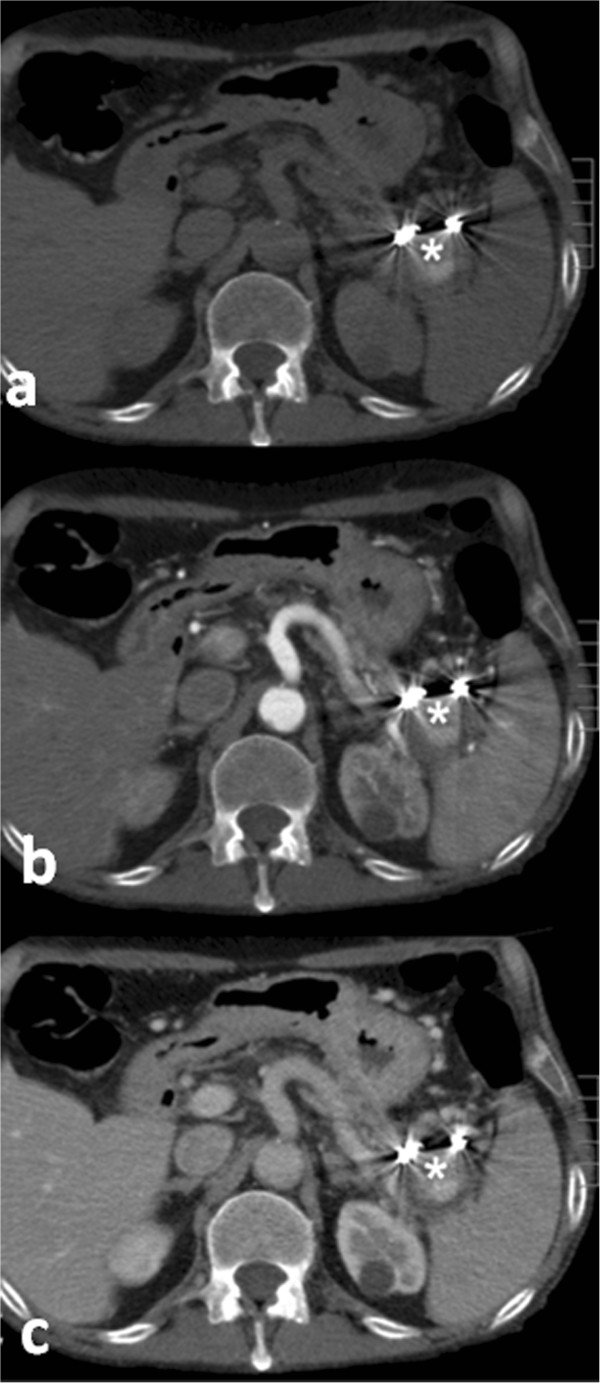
Post-endovascular ligature computed tomography images. Computed tomography performed (a) without intravenous contrast medium administration and (b,c) after administration shows microcoils deployed in the splenic artery, with stagnation of contrast medium inside the pseudocyst (asterisks in a) and exclusion of the pseudoaneurysm in the arterial (b) and venous (c) phase.
